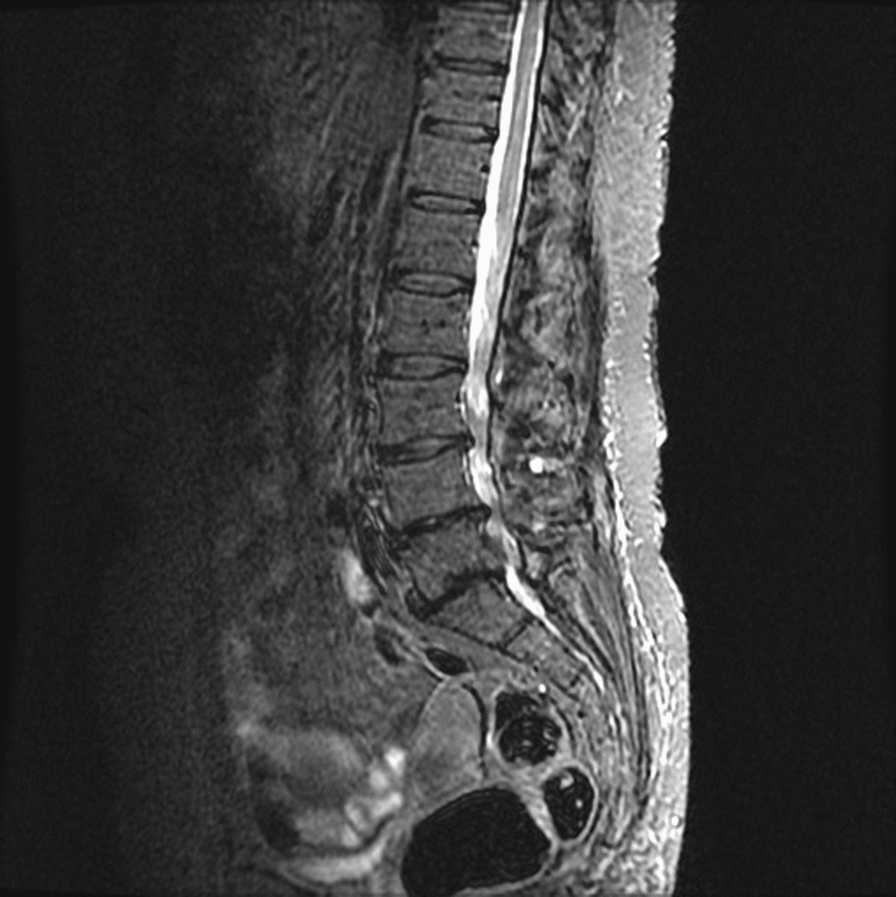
Magnetic resonance imaging of the lumbosacral spine revealing multilevel (L3-S1) degenerative lumbar spinal stenosis
